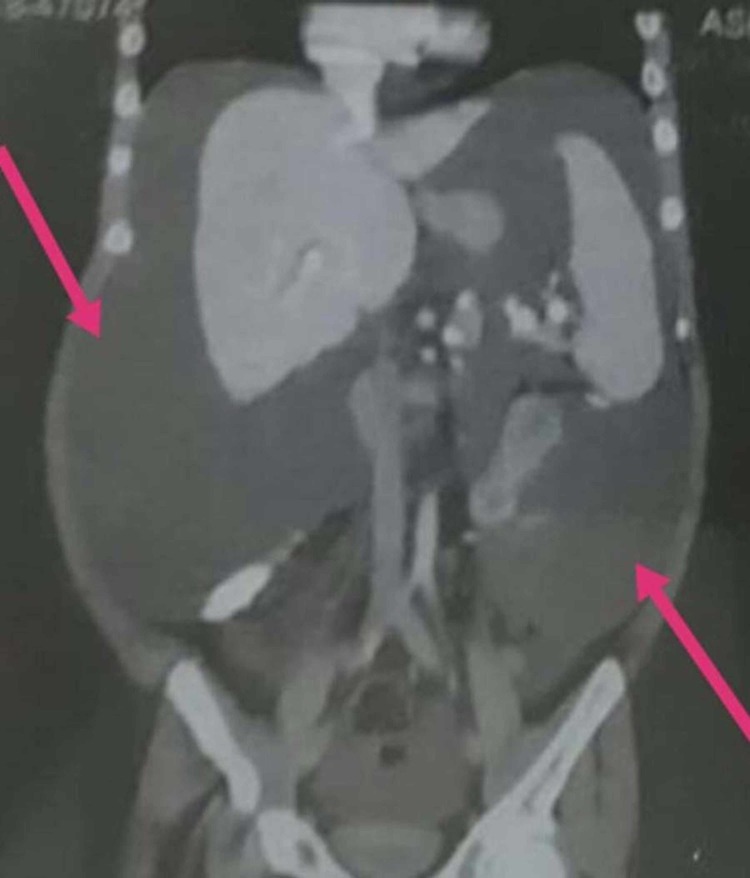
Coronal view CT abdomen showing ascites more marked in pelvic region CT: Computed tomography
